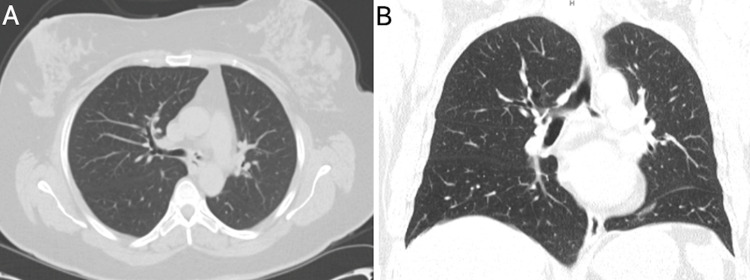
CT of the thorax. (A) Axial cross-section and (B) coronal cross-section of CT of the thorax, showing a narrowing at the level of the proximal left mainstem.
